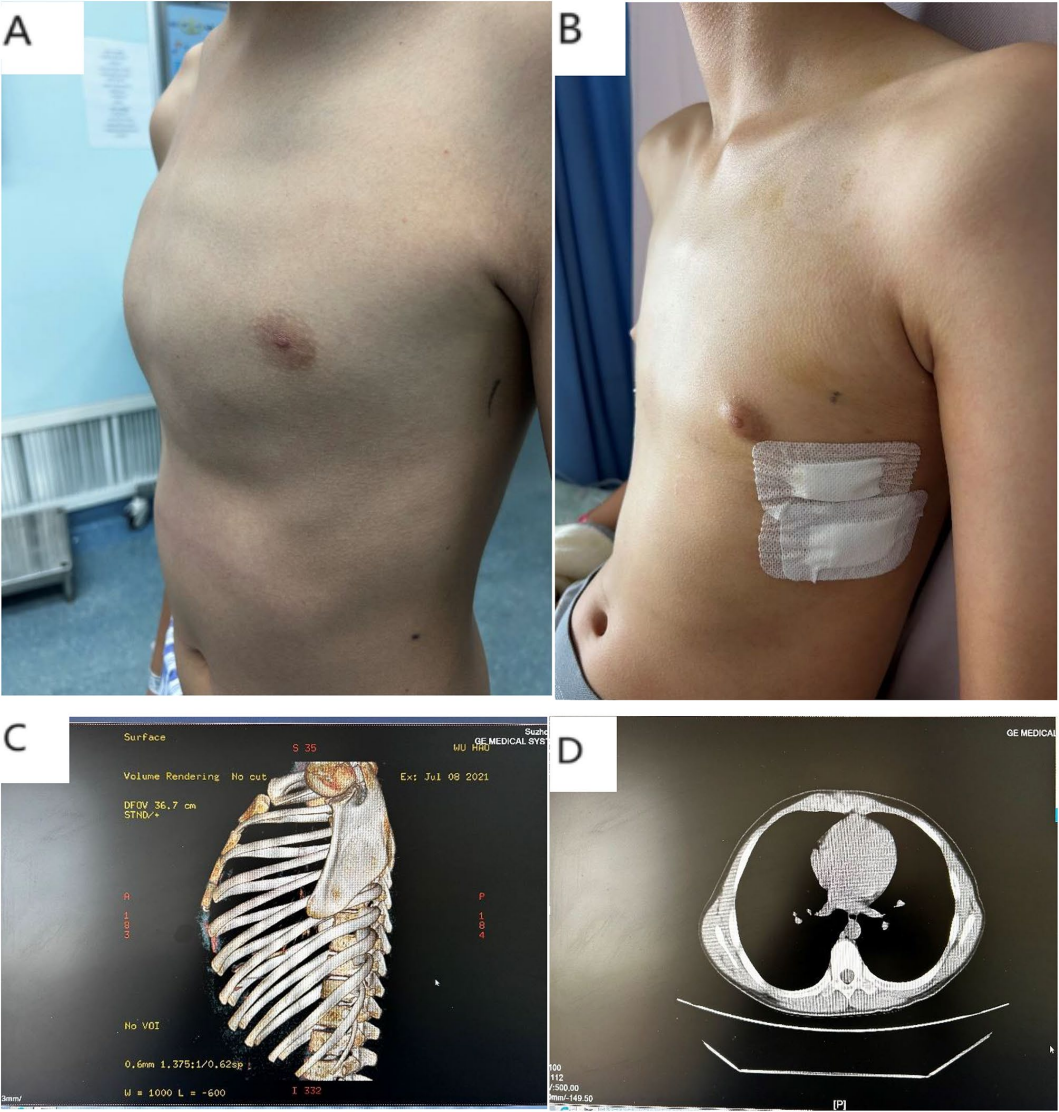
Chest appearance (a: preoperative, b: postoperative )and CT scan (c, d) of symmetrical PC
